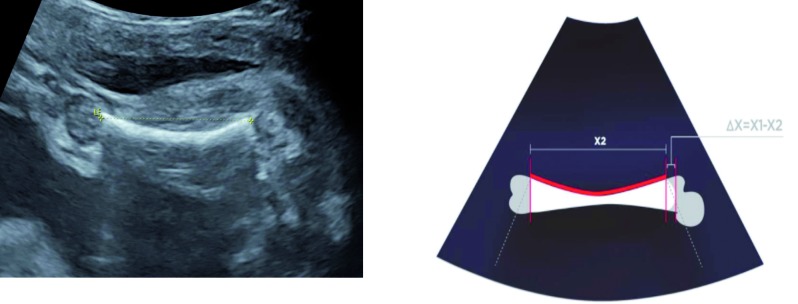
Ultrasound scan of a femur diaphysis measured with an anterior angle. x1 = measure of the diaphysis length in mm.
